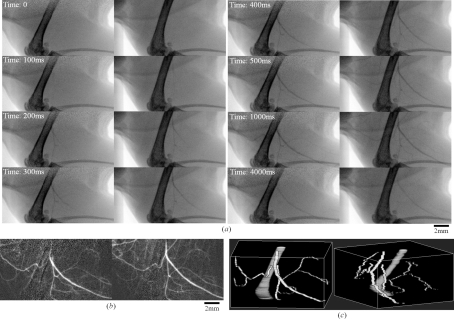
(*a*) X-ray stereo images in angiography. The inset values indicate the elapsed time from the start of the measurement. (*b*) Stereo image of blood vessels obtained by subtracting the stereo image before injection from that after injection (elapsed time:1500 ms). This can be viewed in three dimensions by stereopsis. (*c*) Three-dimensional arrangements of the femur and main blood vessels constructed from X-ray stereo angiography.
